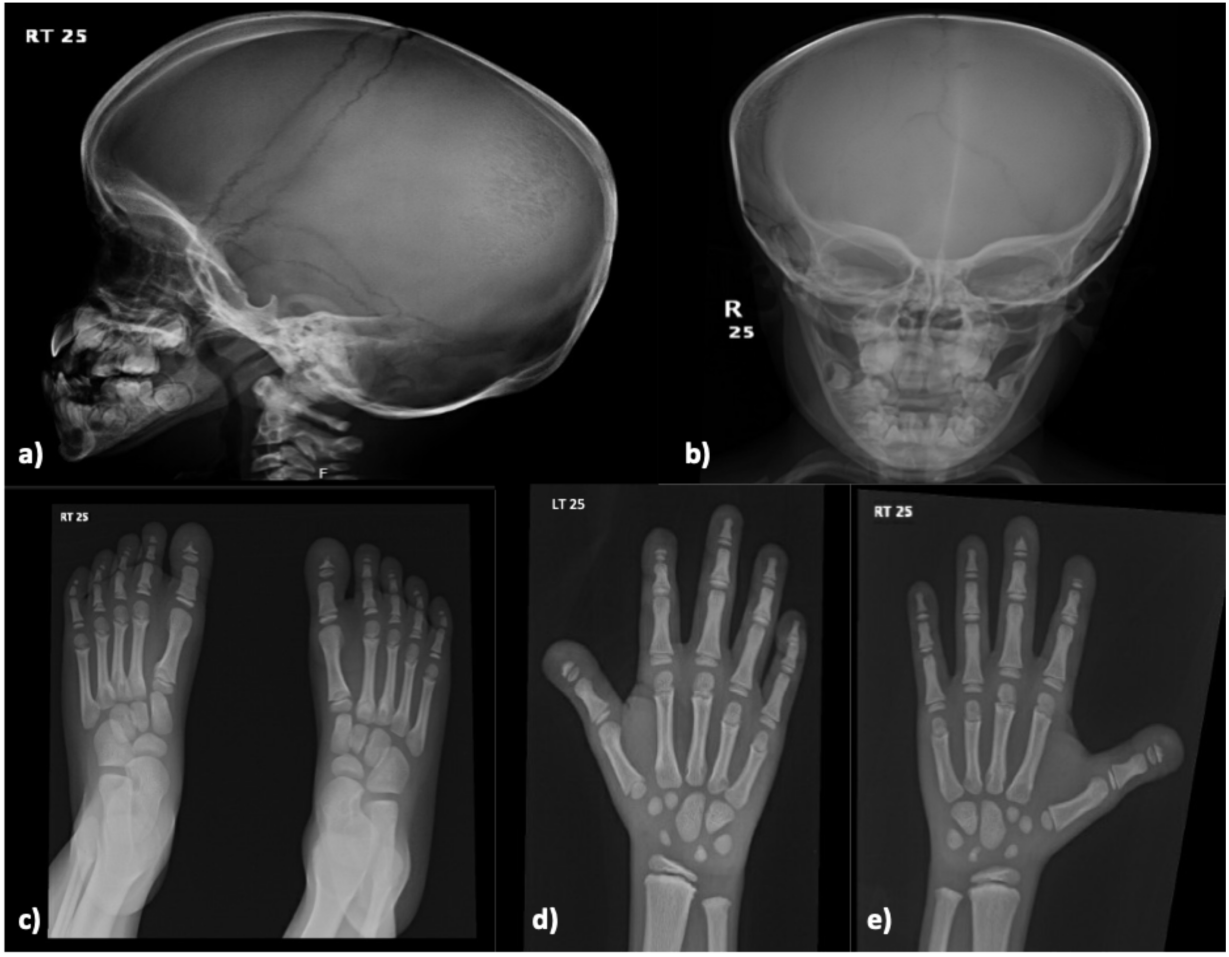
Skeletal X-ray of a male patient done at 8-year-old (Case 8) revealing multiple wormian bones identified in the skull with straightening of the mandibular angle (a, b). There are mild-to-moderate resorption of the distal phalanges of the hands and feet on both sides (c–e). Findings are consistent with Pycnodysostosis.
